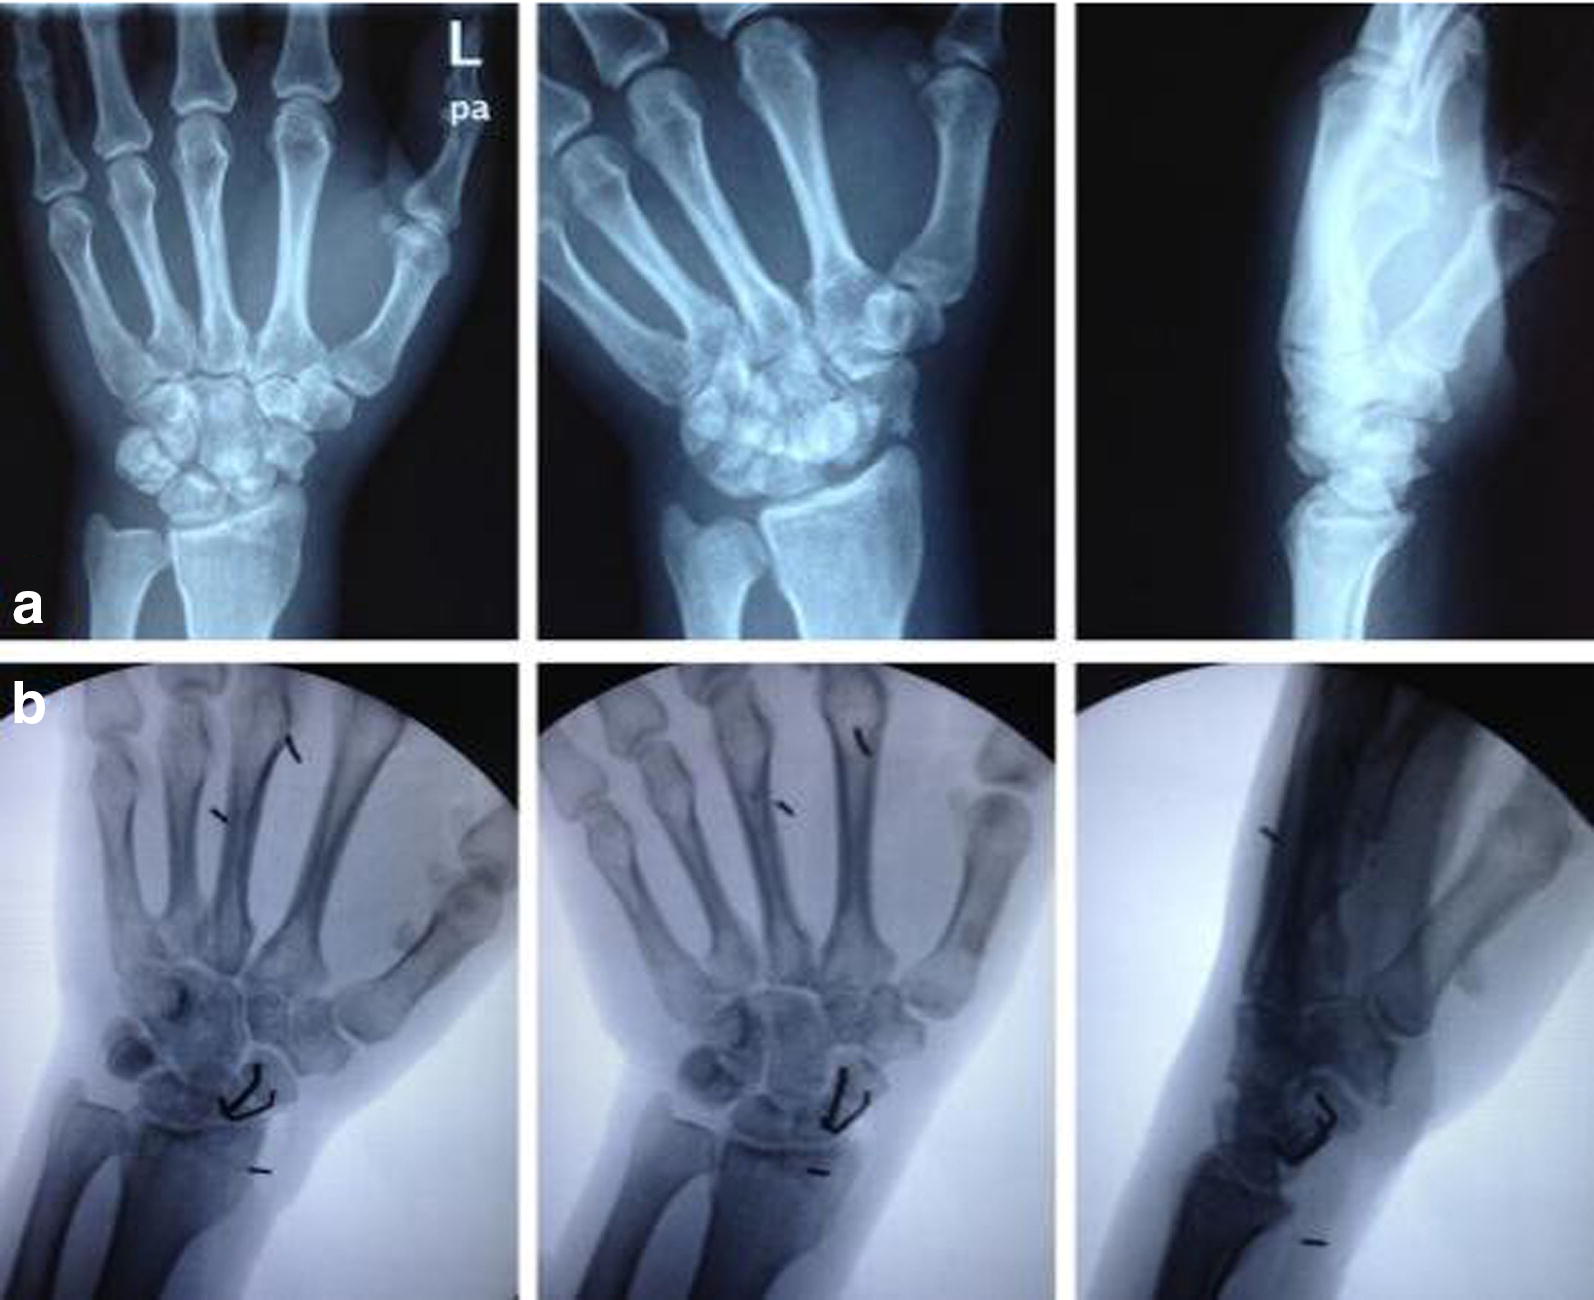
A 32-year-old male patient presented with left wrist pain and discomfort caused by a fall 6 months before followed by cast immobilization. a Preoperative X-ray on anteroposterior, butterfly and lateral views revealed old scaphoid fracture, bone resorption and pseudarthrosis formation at the fracture site. b Postoperative anteroposterior, butterfly and lateral X-ray demonstrated scaphoid fracture reduction, and ASC fixation was in place, and alignment was satisfactory
